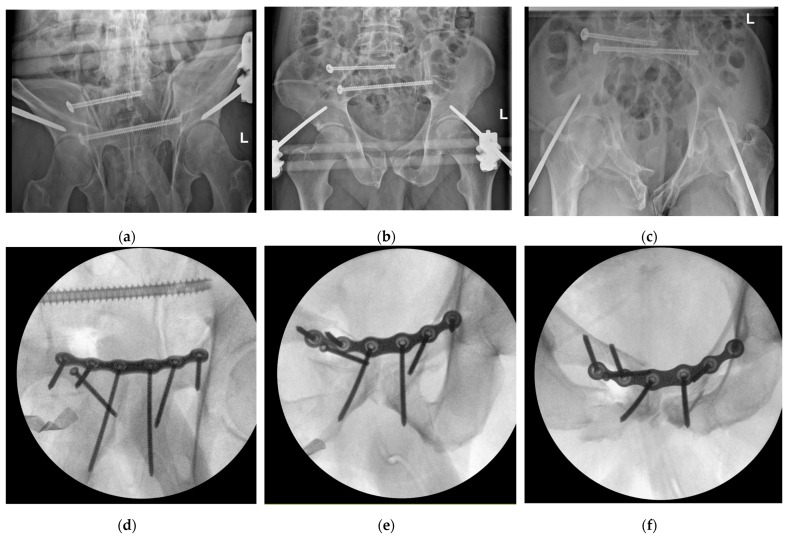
Pre- and intraoperative X-ray imaging documenting the remaining dislocation of the anterior pelvic ring: (a) outlet view after initial reconstruction of the posterior pelvic ring and fixator placement; (b) AP view after initial reconstruction of the posterior pelvic ring and fixator placement; (c) inlet view after initial reconstruction of the posterior pelvic ring and fixator placement; (d) outlet view of intraoperative imaging after repositioning and osteosynthesis; (e) AP view of intraoperative imaging after repositioning and osteosynthesis; and (f) inlet view of intraoperative imaging after repositioning and osteosynthesis.
